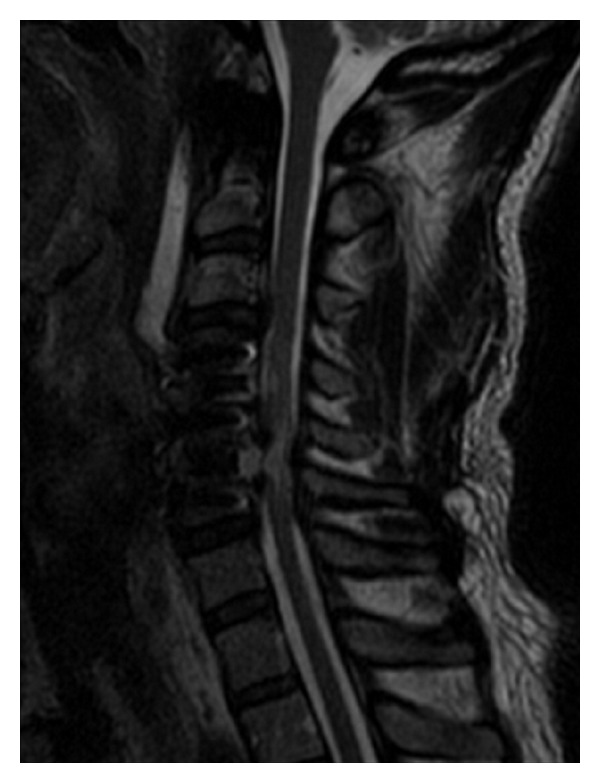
Immediate postoperative MRI after primary C4-5, C5-6 ACDF demonstrates residual C5 compression.
